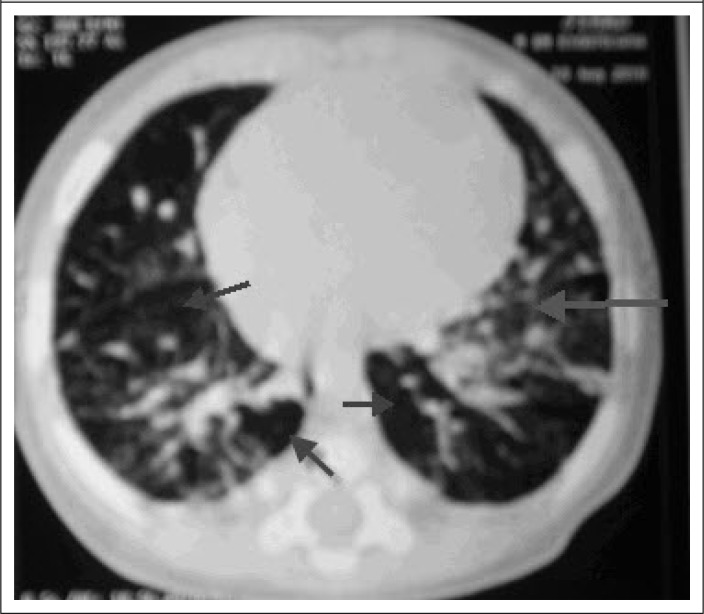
HRCT during the time of diagnosis [ground glass opacities (large arrow) with areas of low attenuation air spaces (air-trapping) (small arrows)]
